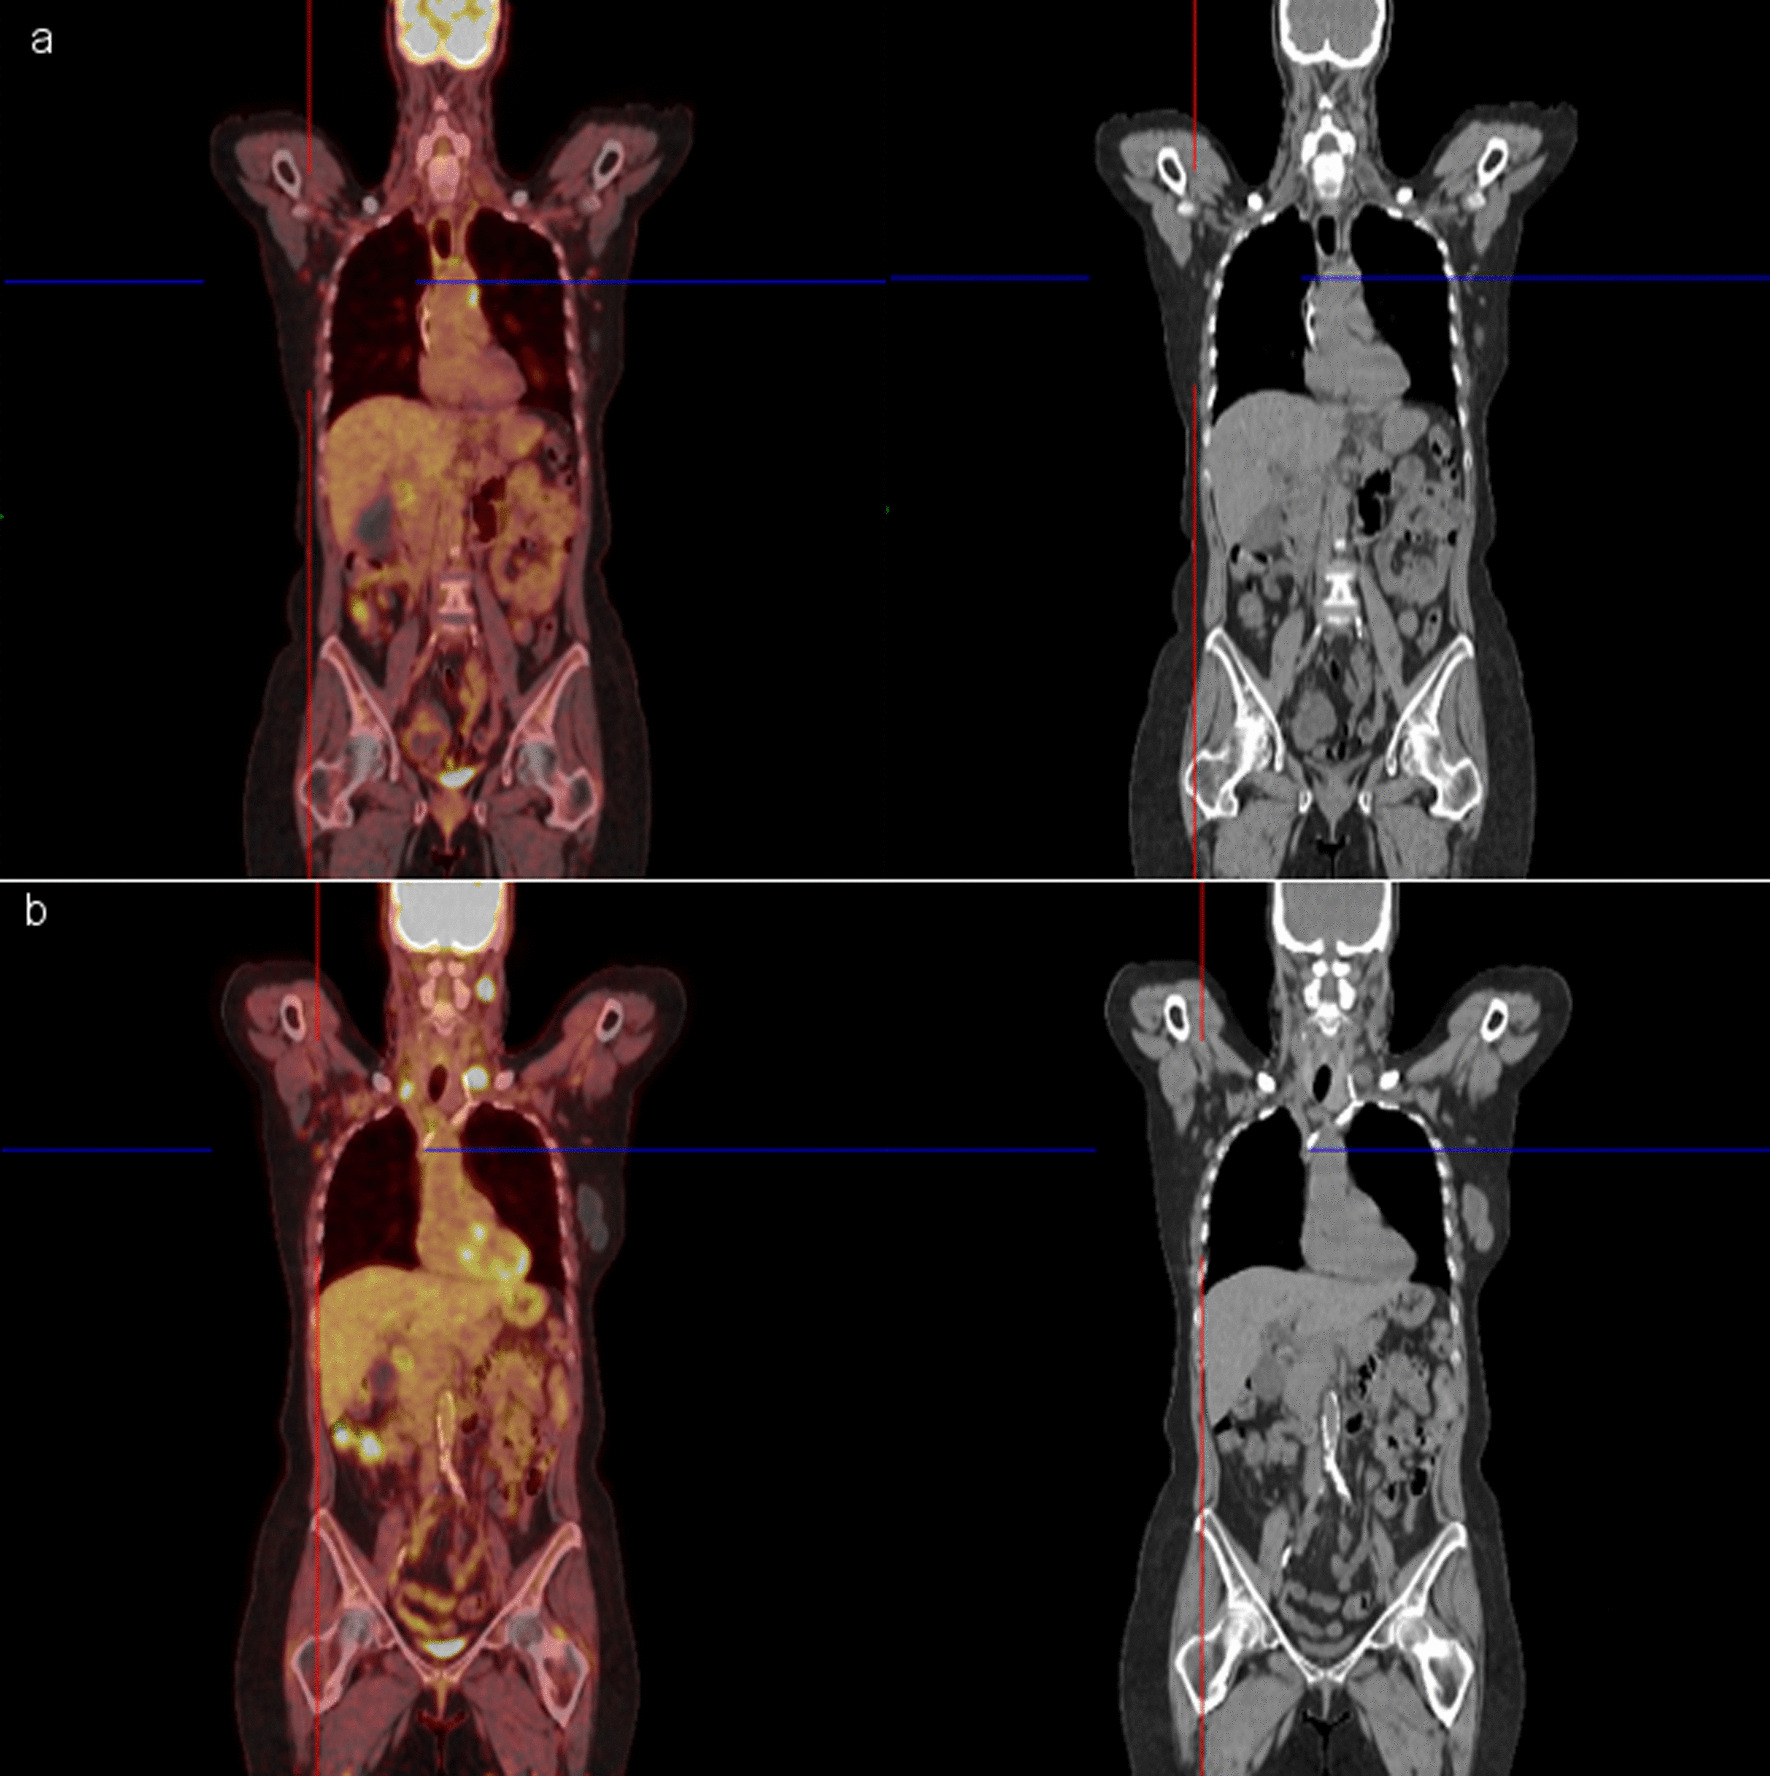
A 61-year-old woman with Stage IV carcinoma of the right lung receiving maintenance immunotherapy had PET–CT scan imaging (a) approximately 15 months prior to receiving her COVID-19 booster vaccination. PET–CT scan imaging of the same woman performed 2 weeks following COVID-19 booster vaccinations (b). Imaging reveals mixed response to treatment with a new left-sided level Xa retroauricular lymph node (1.5 × 1.8 cm, SUVmax 20), interval decrease in the size of the multilevel bilateral subcentimeter cervical chain lymph nodes without FDG uptake, and a right-sided level III lymph node measuring 1 cm with max SUV 8.0 (previously 8 mm and SUVmax 8.5). Interval increase in the size of the hypermetabolic level IVa lymph node measuring 1.8 cm with SUVmax 14.8 (previously 8 mm, SUV 5.3); and new left supraclavicular hypermetabolic lymph node measuring 8 mm with SUVmax 14.6
